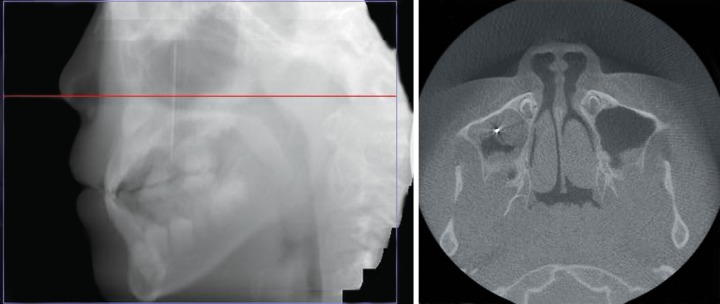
CBCT scan revealed a hyperdense image within the right maxillary sinus, and the sinusal mucosa was thicker than the normal left side
